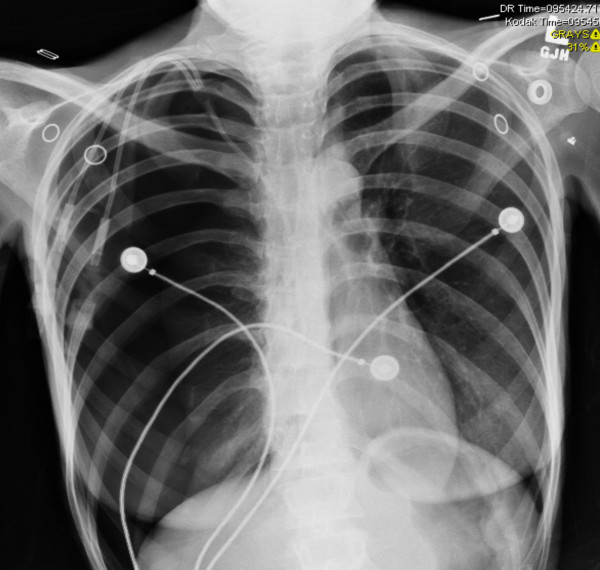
Chest x-ray demonstrating large right pneumothorax on the day after placement of the central venous catheter.
